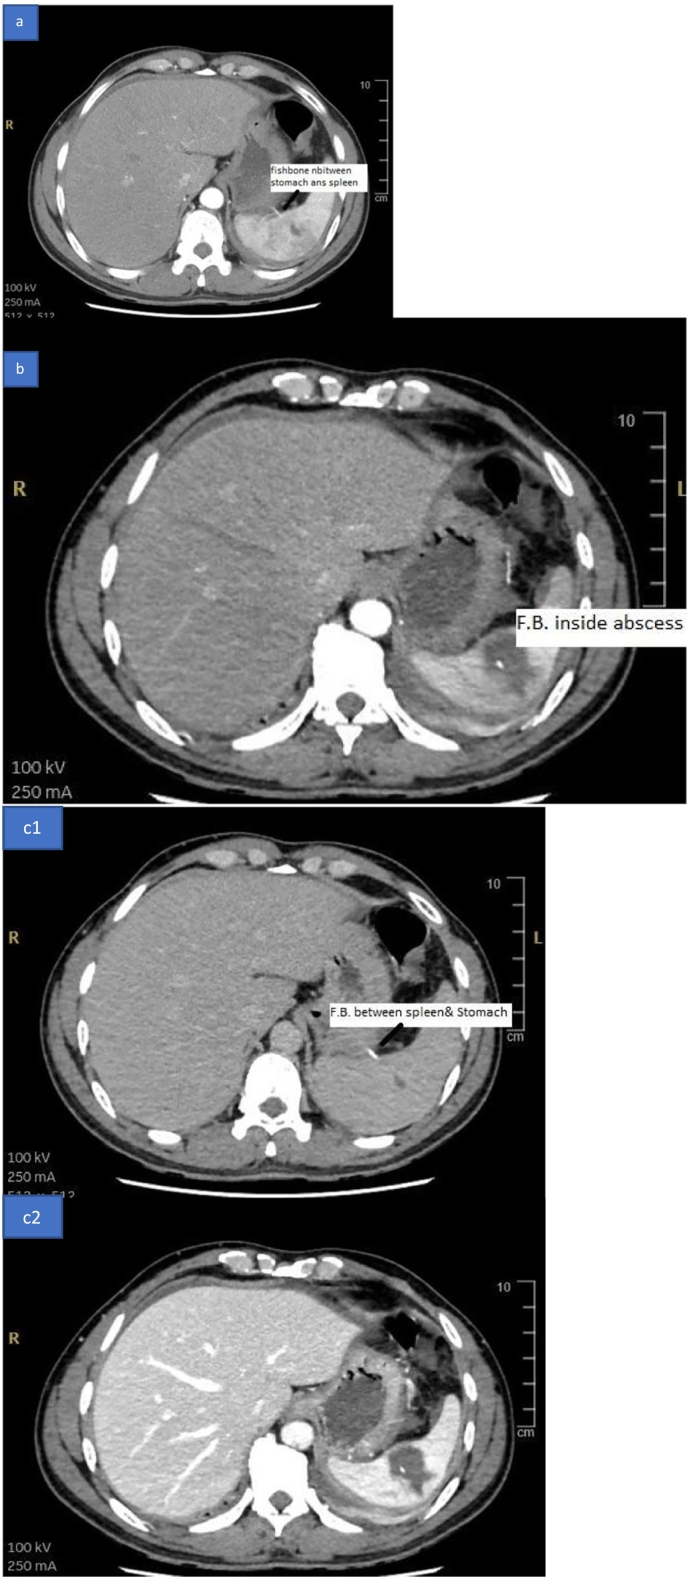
CT scan of the abdomen showing a & c1: Foreign body between stomach and spleen, b & c2: foreign body inside splenic abscess.
